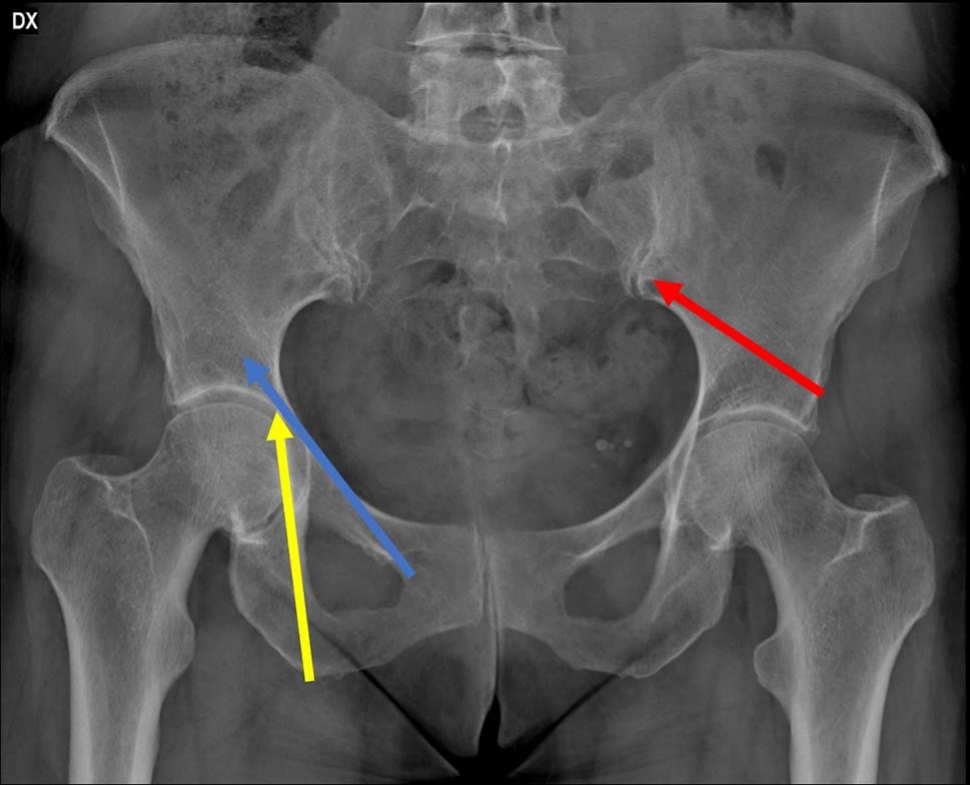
Antero-posterior standard X-ray, showing the three corridors for the percutaneous fixation of the acetabular fractures. In Blue, the iliac-pubic corridor (in retrograde fashion) for the anterior column. In Yellow, the iliac-ischiatic corridor for the fixation for the posterior column. In Red, the dome corridor
